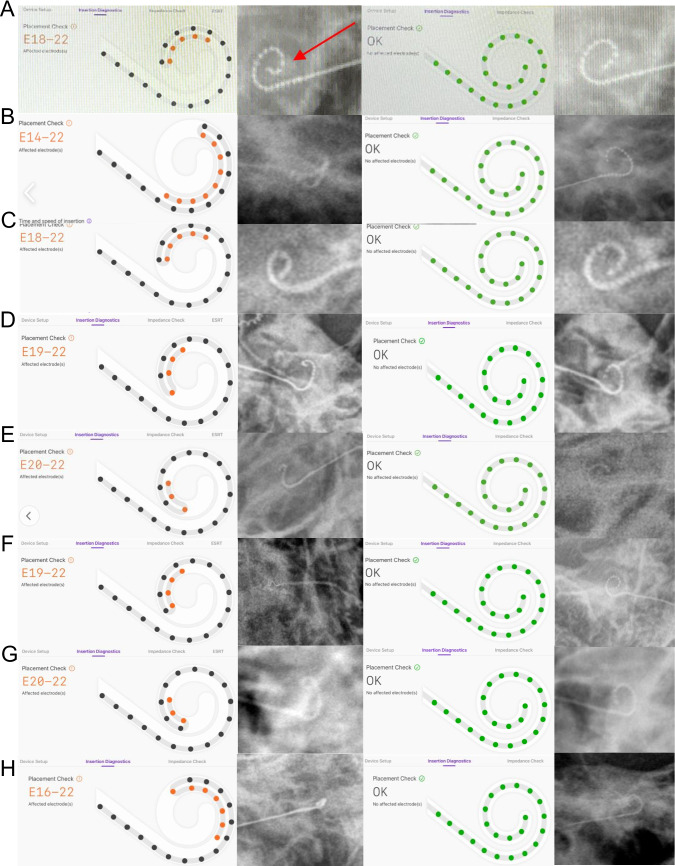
A tip fold-over (TFO) detected by SmartNav system. Eight cases (A to H) are demonstrated. A TFO was detected in the initial SmartNav system (first panels, A to H), which is confirmed by X-ray images (second panels, A to H). After reloading and reinsertion, correct placement of electrodes within the cochlea is verified by both SmartNav (third panels, A to H) and X-ray images (fourth panels, A to H). Note that a tiny kinking of the distal electrode (red arrow, second panel of A), which might have been overlooked when relying solely on X-ray for detection, is detected by SmartNav system (first panel of A). For the second panel of Fig. 1F, we acknowledge that the resolution is suboptimal. To enhance visual clarity, particularly in the electrode channel regions, we have added white dots to mark the positions of individual channels
